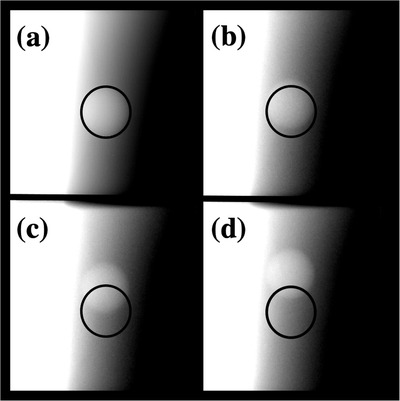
Images of a radiographic target moving at constant velocity were used to measure end‐to‐end latency of the gating system. The reference position was contoured and overlaid on each of the images. Images used to measure beam‐on latency are shown for target velocities of (a) 0 mm per second (reference position), (b) 1.5 mm per second, (c) 4.5 mm per second, and (d) 7.5 mm per second.
